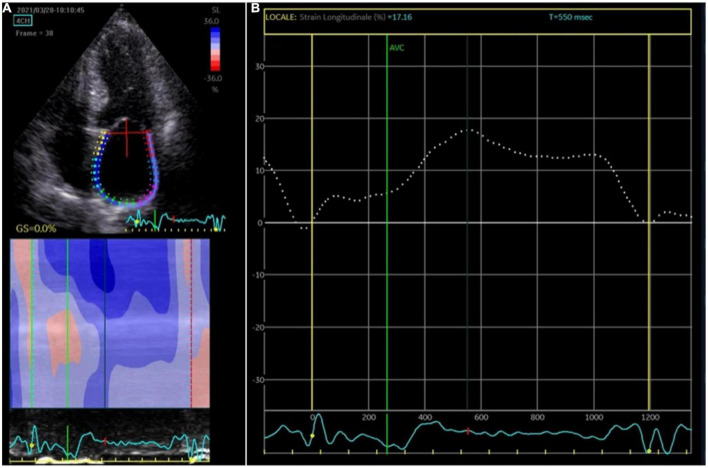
Atrial strain measurement. (A) The apical four-chamber view was utilized for the strain measurements of the left atrium (LA); the edge of the LA endocardium was manually traced. (B) The software generated tracings based on the 2D strain of LA. The mean deformation (strain) is expressed in percentage and calculated by the software.
